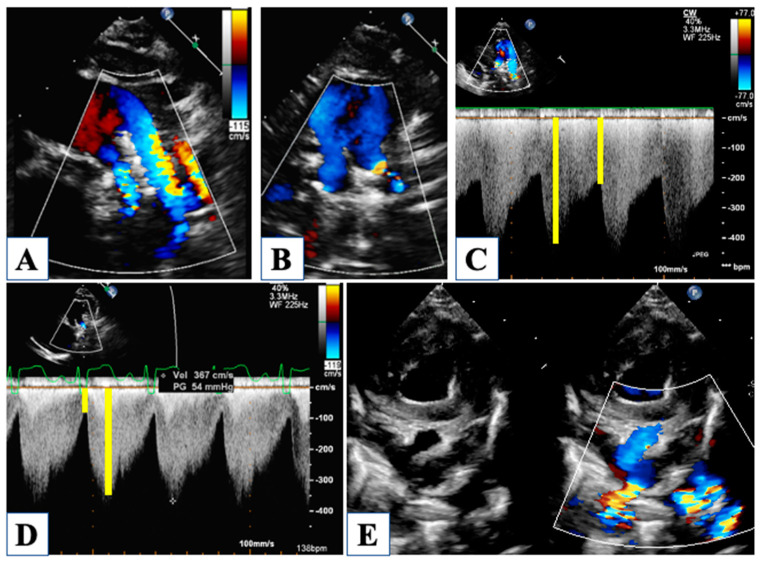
Pulmonary branches banding. The right pulmonary artery is well visible below the ductal stent in Panel (A), while both branches may be imaged at same time from a high parasternal short axis (Panel (B,E)) with evident aliasing on color Doppler. Continuous-wave Doppler through the banded branches shows sisto-diastolic high-velocity flow, in panel (C) a normal Doppler pattern, in panel (D) an example of high pulsatility index (high systolic velocity with low diastolic velocity) and steep systolic slope consistent with high pulmonary pressures.
